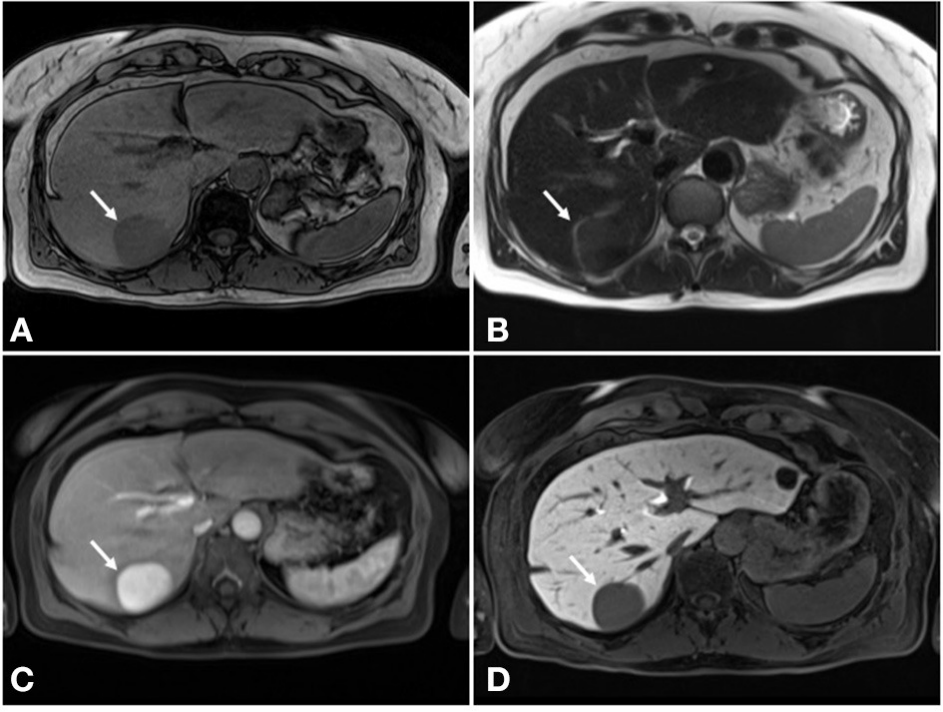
MRI of the liver. (A) Hypointense signal on T1-weighted sequences. (B) Hyperintense signal on T2-weighted sequences. (C) Complete homogenous enhancement in post-contrast arterial phase. (D) Hypointense signal in hepatobiliary delayed phase.
